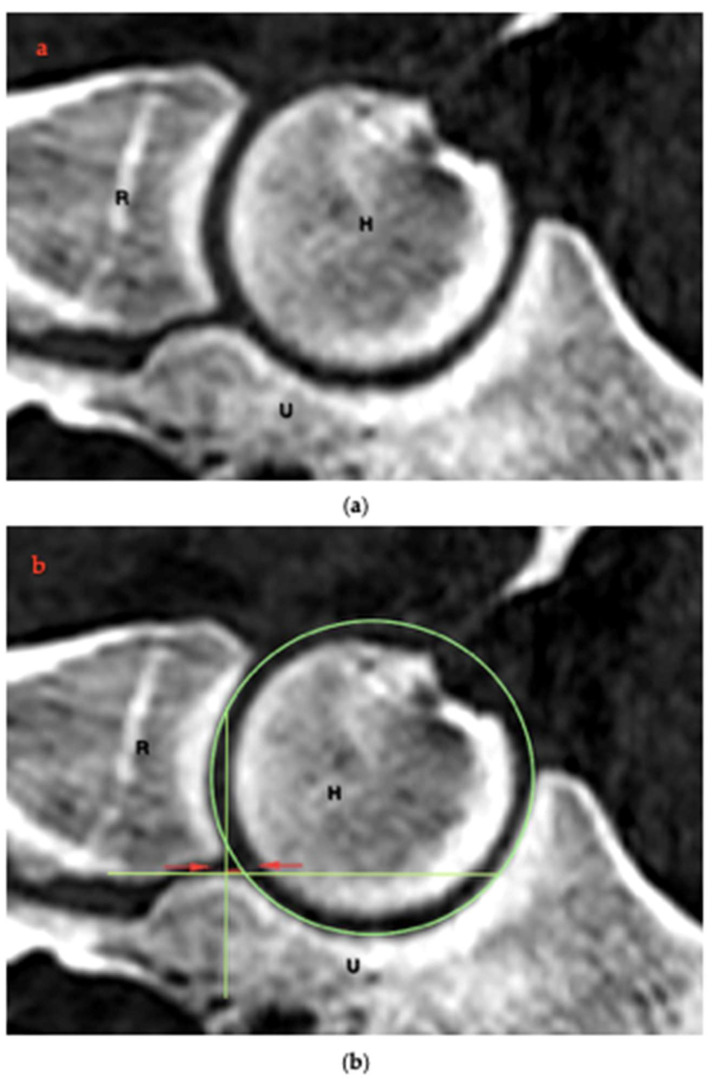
(a,b) Sagittal CT image of the elbow (a) showing the RUI BMC ((b), the red line between red arrows) using a circular graphical construct (green circle and green lines marking the bone surface of the radius and ulna). H: humerus, R: radius, U: ulna.
